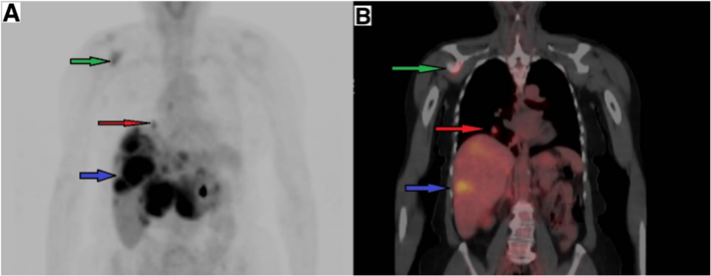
A Position emission tomography scan in coronal view shows metastasis to right scapula (green arrow), a 1.7cm right lower lobe lung nodule (red arrow) and multiple liver metastases (blue arrow). B Position emission tomography-computed tomography fusion scan in coronal view shows metastasis to right scapula (green arrow), a 1.7cm right lower lobe lung nodule (red arrow) and multiple liver metastases (blue arrow).
